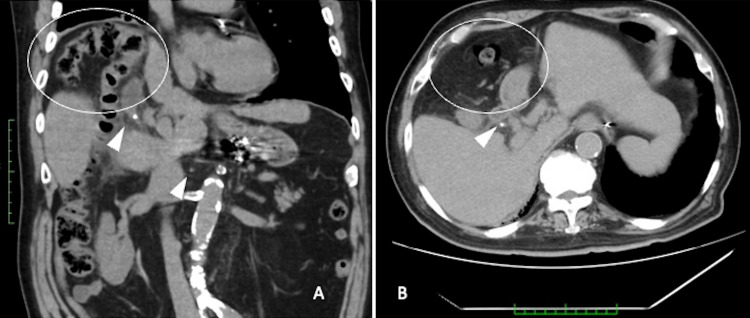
Case 2: Computed tomography (CT). Computed tomography (CT) revealed a gallstone impacted in the cystic duct (A, arrowhead). Additionally, the transverse colon was interposed between the diaphragm and the liver and was in close contact with the gallbladder (A and B, circles).
